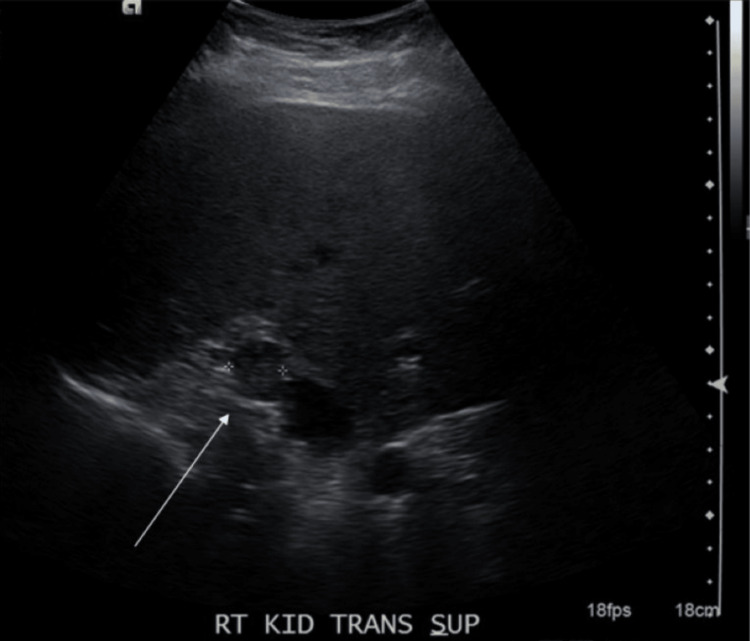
Kidney US displaying a questionable right kidney mass US: ultrasound
